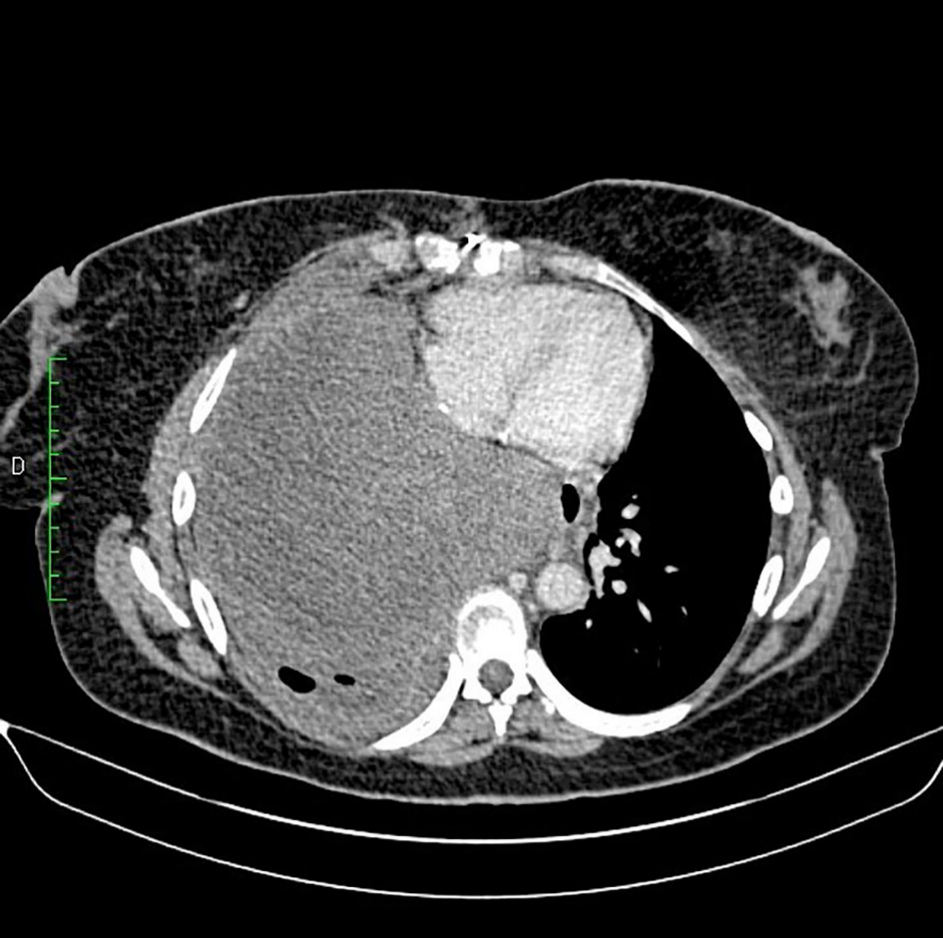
CT (coronal reconstruction): huge relapse of mediastinal mass with cardiac and tracheal compression.
